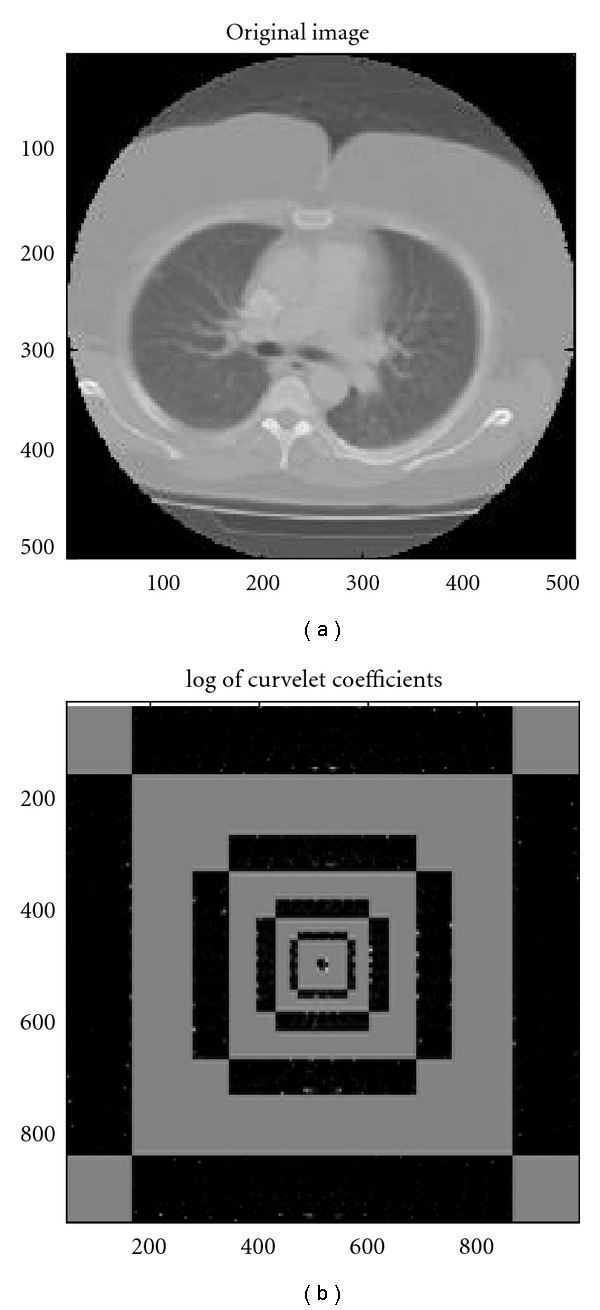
Clinical slice for the human chest from a CT scanner in spatial (a) and curvelet coefficients (b).
